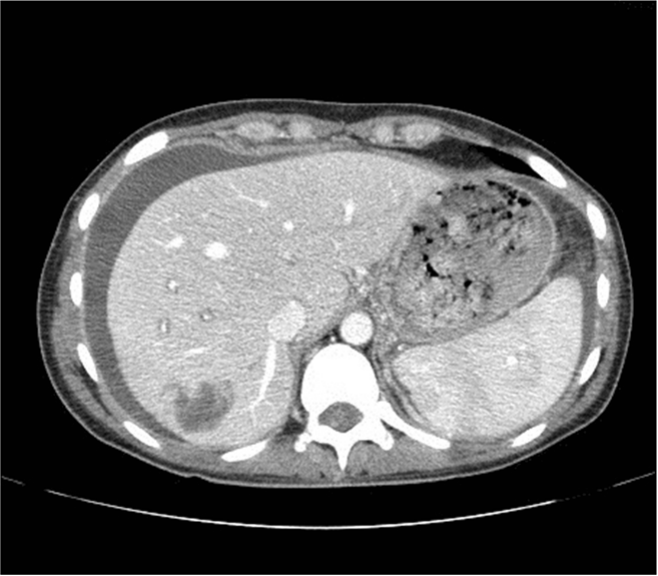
Abdominal computed tomography image showing large right liver lobe abscess accompanied by perihepatic and interintestinal biloma.
